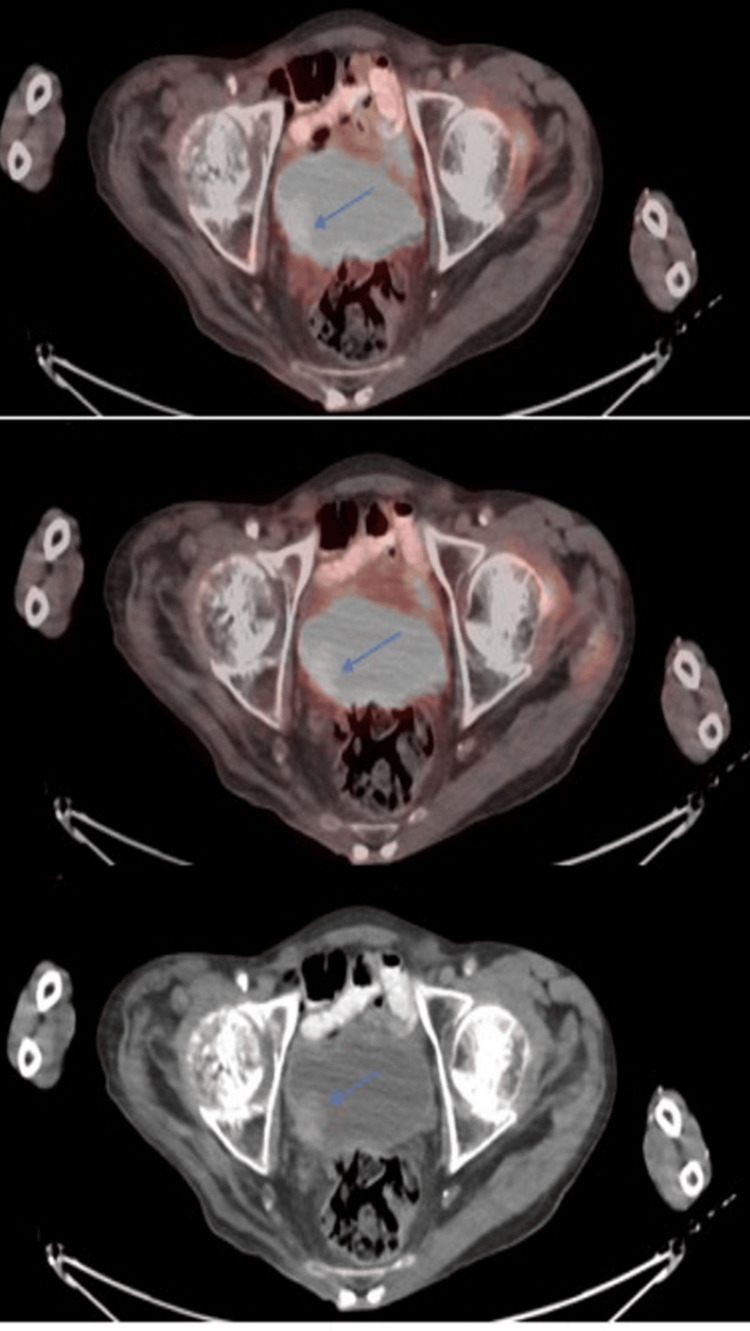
Focal hypermetabolic mass in the urinary bladder on PET-CT.
